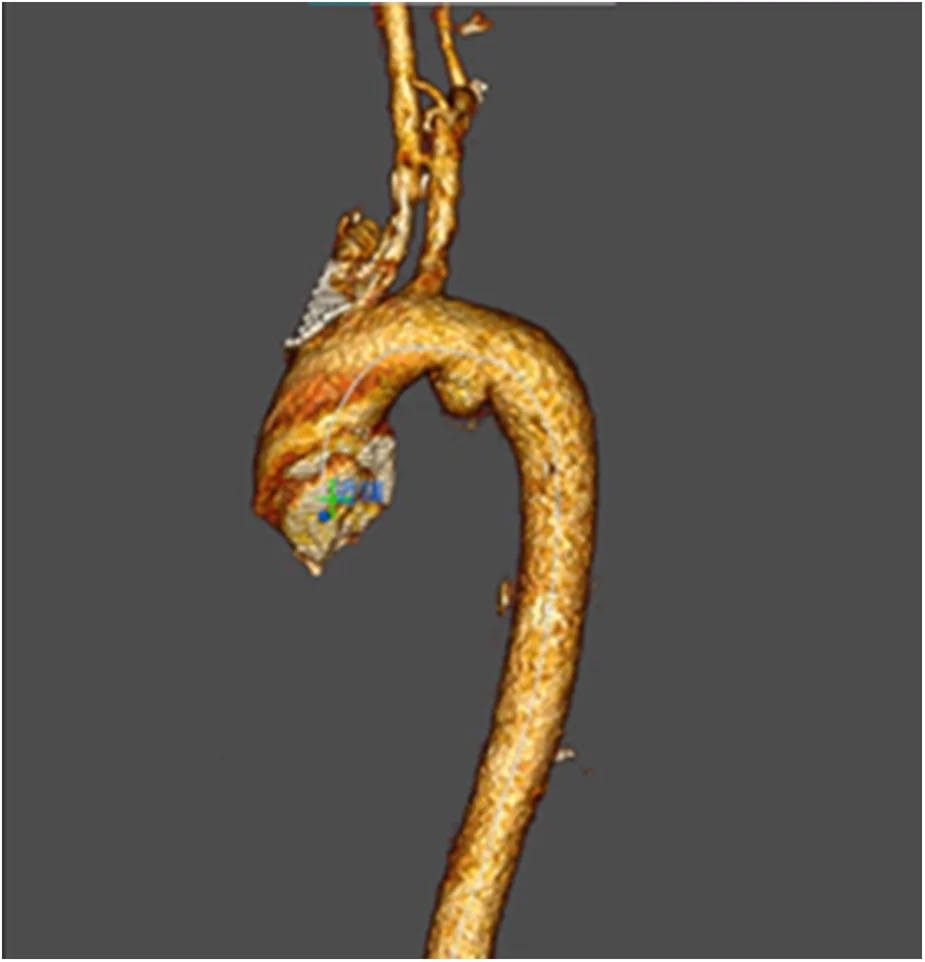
Thoracic aortic CTA demonstrating a pseudoaneurysm at the aortic isthmus.
